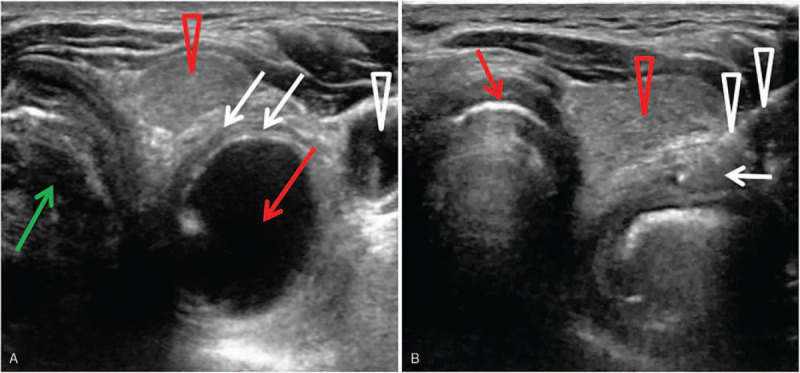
(A) An ultrasound showed that the dilated balloon of a Foley catheter was blocked and situated below the CP muscle before injection. White arrow: the CP muscle. White sharp triangle: The left common carotid artery. Red arrow: The inflated balloon below the CP muscle. Red sharp triangle: The thyroid. Green arrow: The windpipe. (B) Ultrasound-guided injection to the CP muscle and sagittal view for in-plane approach. White arrow: The CP muscle. White sharp triangle: The advancement of the needle (diameter 0.51 mm, length 60 mm). Red arrow: The thyroid cartilage. Red sharp triangle: The thyroid.
